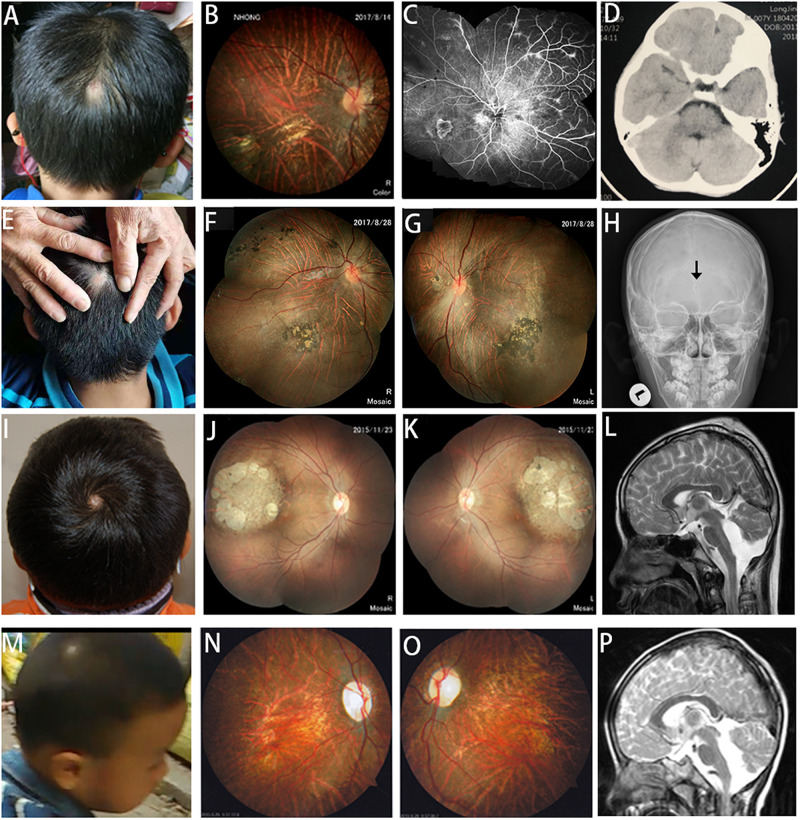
Scalp defects, fundus changes, and neuroimaging in patients with *COL18A1* and *LAMA1* mutations. (A,E) Alopecia areata appears in the midline of the occipital region of patients with *COL18A1* mutations. (I,M) Alopecia areata appears in the midline of the parietal region (in the center of hair whorl) in patients with *LAMA1* mutations. (B,C,F,G,J,K,N,O) In addition to the typical high myopia fundus leopard-like pattern, dysplasia of the papilla, and macular degeneration, characteristic fundus degeneration can be observed for all patients including optic degeneration, chorioretinal sclerosis and atrophy, foveal hypoplasia, atrophic patch with extra bone spicule accumulation, and loss of pigmentation temporal to the macula. (C) Fluorescein angiograms reveal vascular leakage in patient 14410. (D) MRI reveals a normal brain image for patient 14410. (H) Black arrow indicates a small hole in the occipital region of patient 14518 by X-ray. (L,P) Cerebellar dysplasia and obvious enlargement of the fourth ventricle are observed in patients 5176 and 7856.
